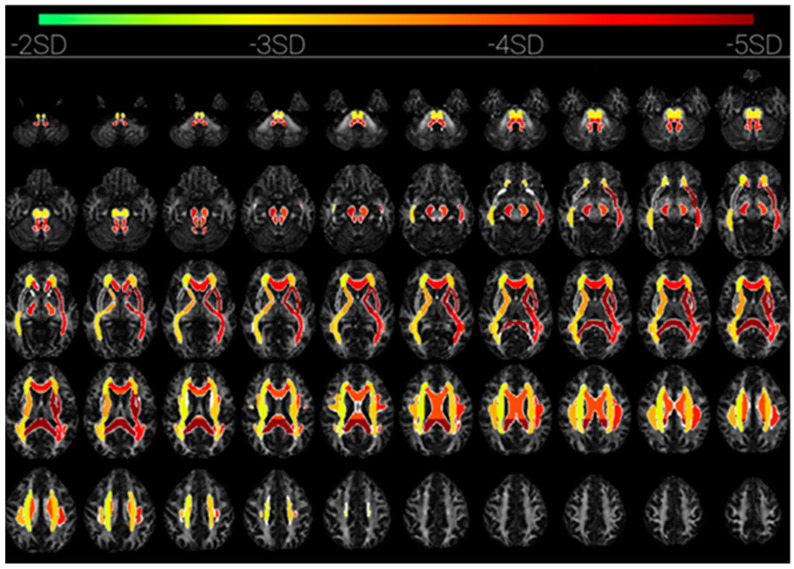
Brain MRI, Diffusion Tensor Imaging DTI sequence: mean fractional anisotropy within regions of interest expressed as number of standard deviations of the normal measurement in healthy subjects (software version 1.1, from brainQuant, Paris, France) [15].
